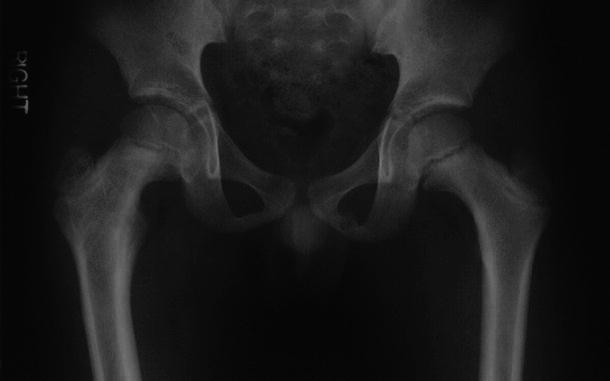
At two-year follow up, radiographs show union of the femoral neck fracture without avascular necrosis of the femoral head
